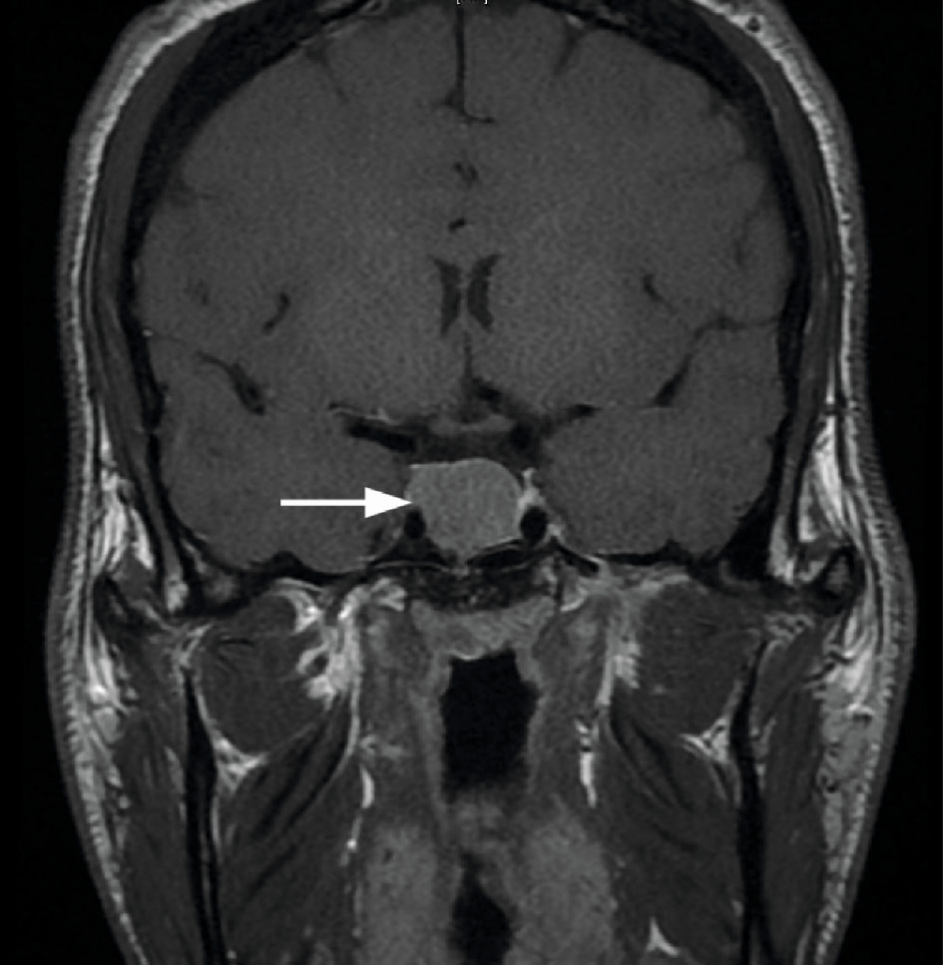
Magnetic resonance imaging (MRI). The sella was expanded by a macroadenoma (white arrow) with mild contrast enhancement.
